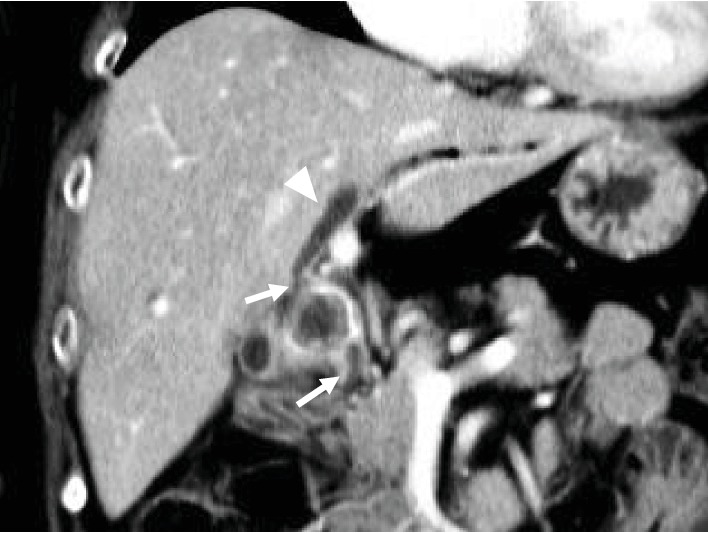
Abdominal CT scan on admission. The CT scan revealed that the gallbladder stone was obstructing the common bile duct (arrows) with dilatation of the intrahepatic duct (arrowhead).
